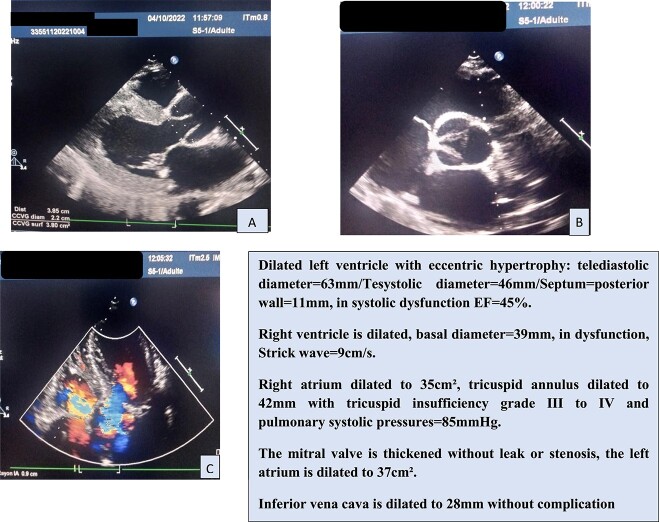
(A–C) Transthoracic echocardiogrphy sections.
